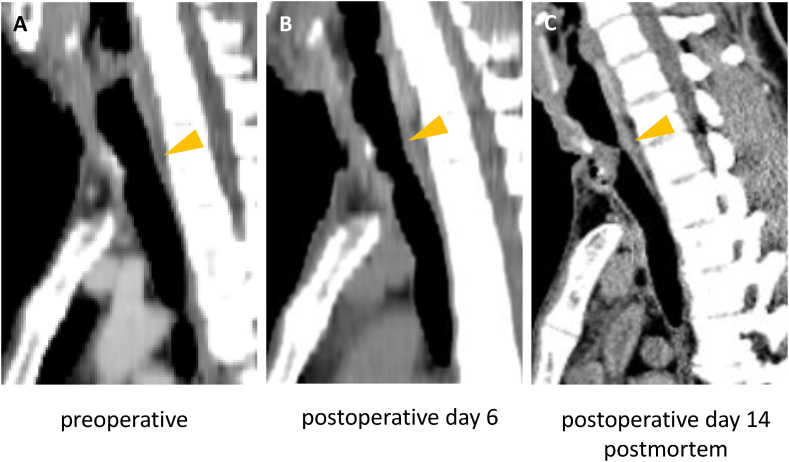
A-B. CT images of sagittal section of the trachea. A. preoperation, B. post operation after decannulation of tracheostomy (postoperative day 6), C. postmortem (postoperative day 14). Stenosis of trachea (arrowhead) was observed since preoperation but progressed after decannulation of tracheostomy.
